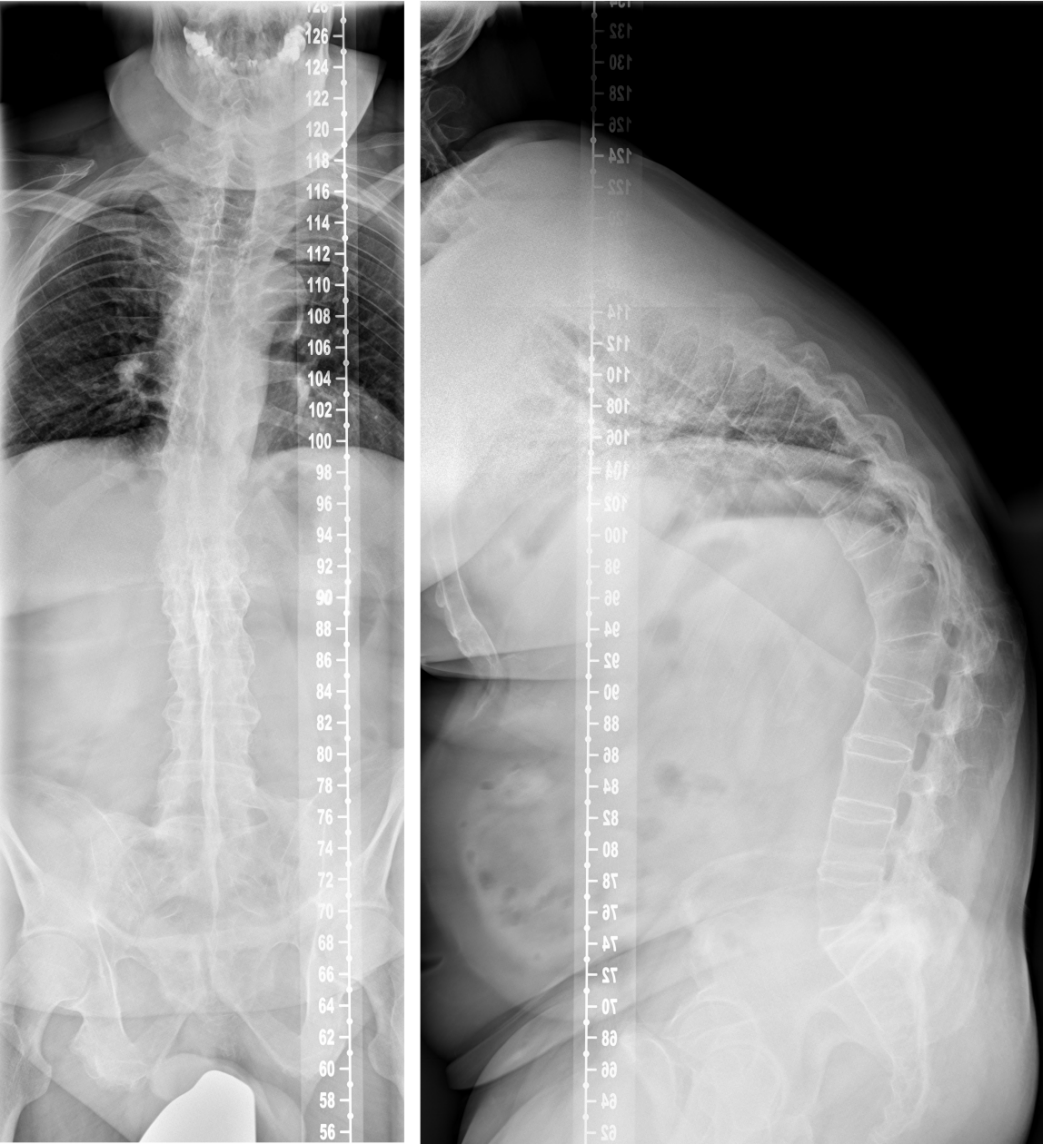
Conventional X-rays of the whole spine in two planes in standing position (ap-view left and lateral view right) revealing the ossification and the kyphotic deformity of the whole spine with sagittal imbalance as well as the reduced bone mineral density.
